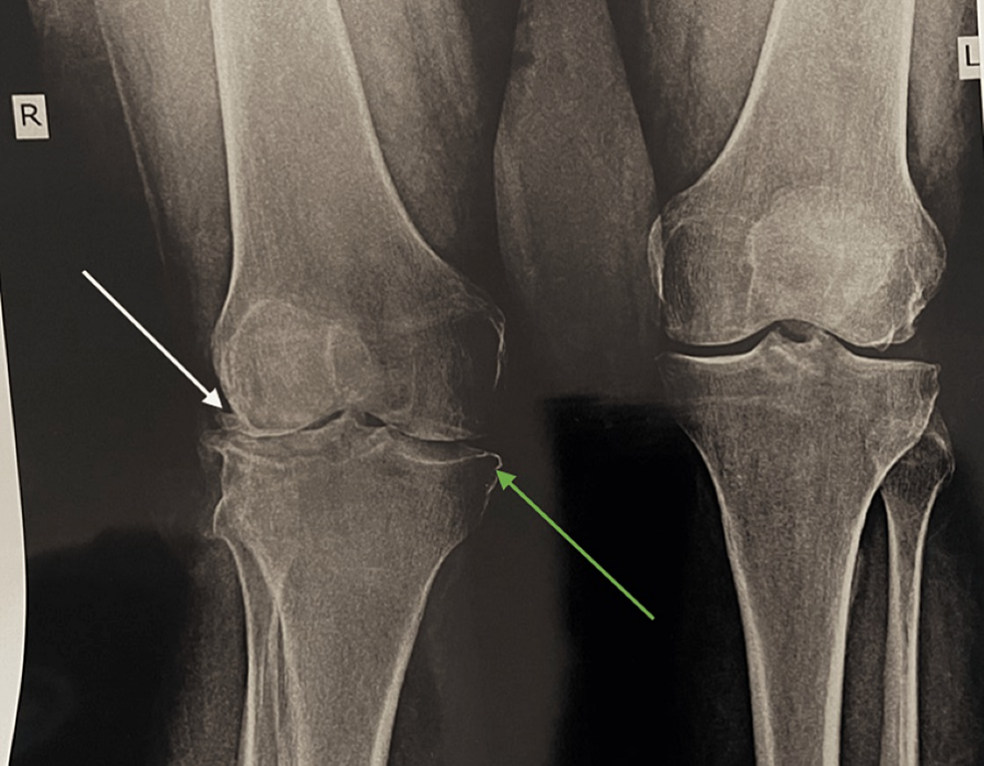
X-ray anteroposterior (AP) view It shows osteoarthritis of knee with joint space narrowing, sclerosis of bone, and multiple osteophyte formation representing grade three of knee osteoarthritis (OA) according to Kellgren- Lawrence scale.
